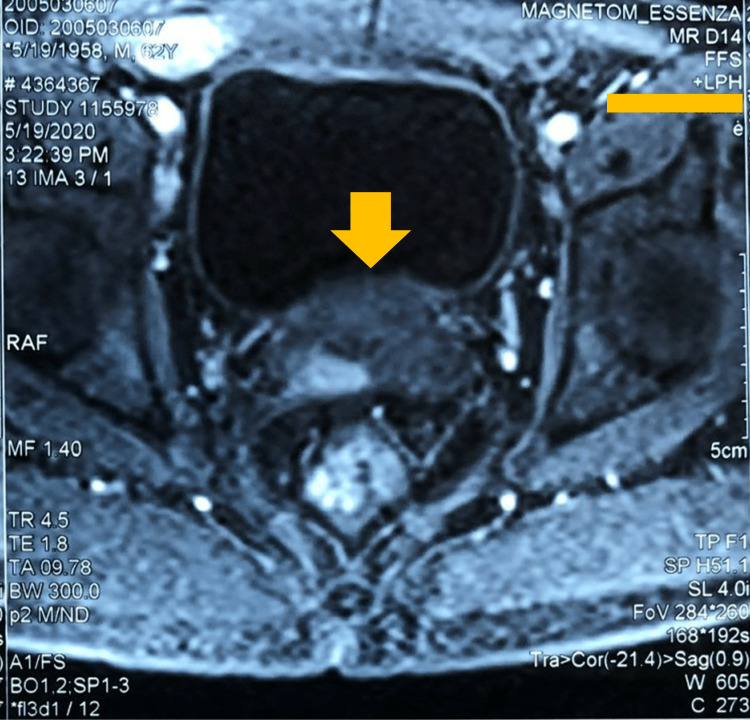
Magnetic resonance imaging showing an enlarged prostate with increasing signal of the parenchyma on T2-weighted imaging.
